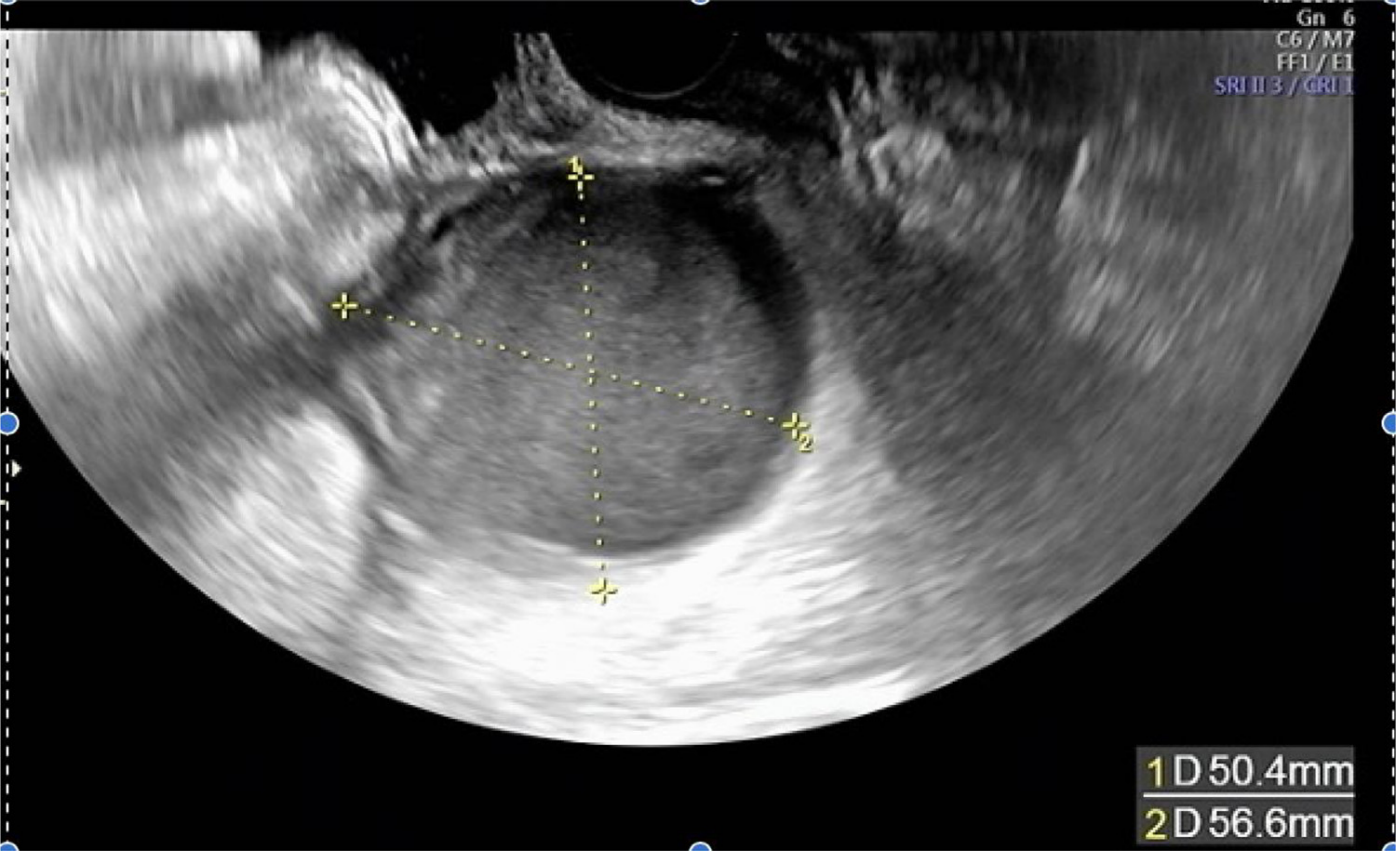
Transvaginal ultrasound showing a 5-cm ovarian tumor (indicated by the yellow lines).
